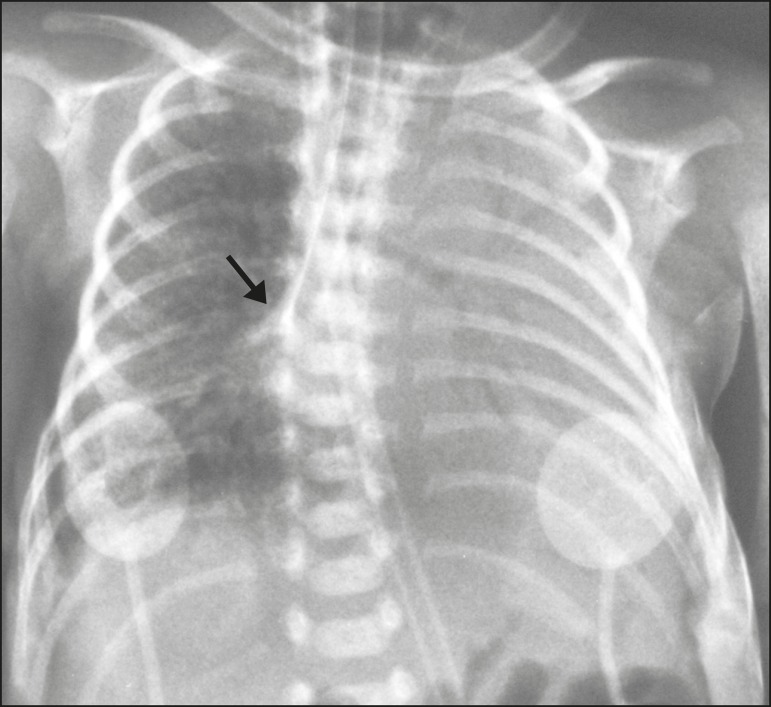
Chest X-ray of a neonate born at 29 weeks of gestation with a birth
